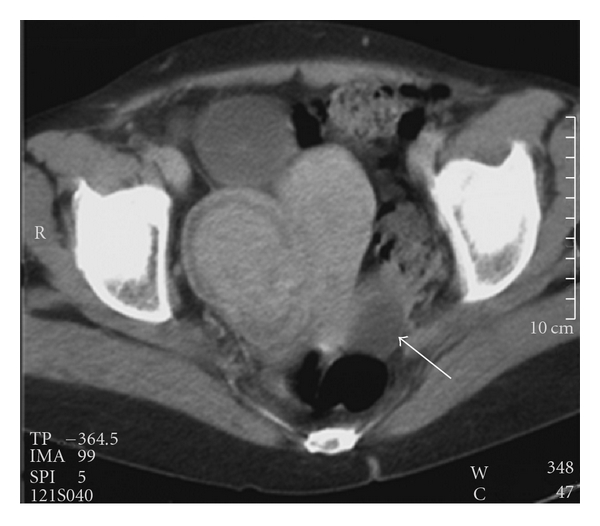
Contrast-enhanced CT of the pelvis showing a large myoma on the right side of the uterus. Note the cyst-like lesion in the left ovarium, without intraluminal nodules (arrow).
